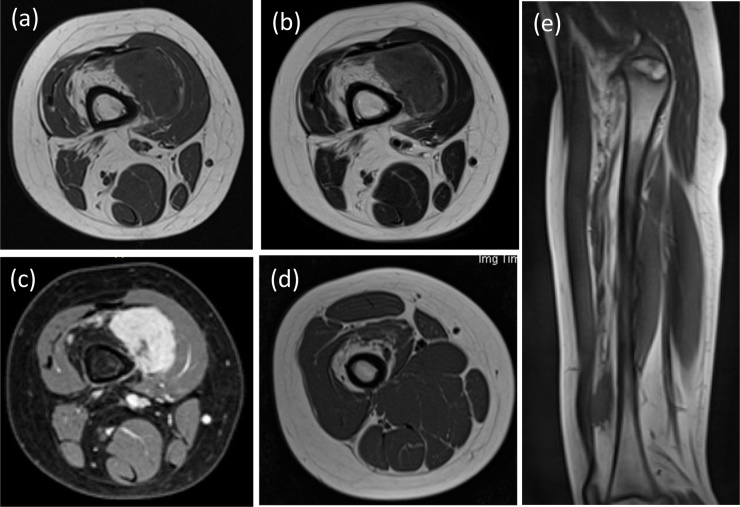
Pre-operative magnetic resonance image of the right femur. The lesion was located beneath the vastus medialis muscle, and the vastus intermedius muscle showed fatty degeneration. Axial view of the right distal femur on (a) T1-weighted imaging, (b) T2-weighted imaging, and (c) gadolinium-enhanced, T1-weighted, fat-suppressed imaging. (d) Axial view of the right proximal femur and (e) sagittal view of the right femur on T1-weighted imaging show that fatty degeneration stretched the full length of the vastus intermedius muscle.
